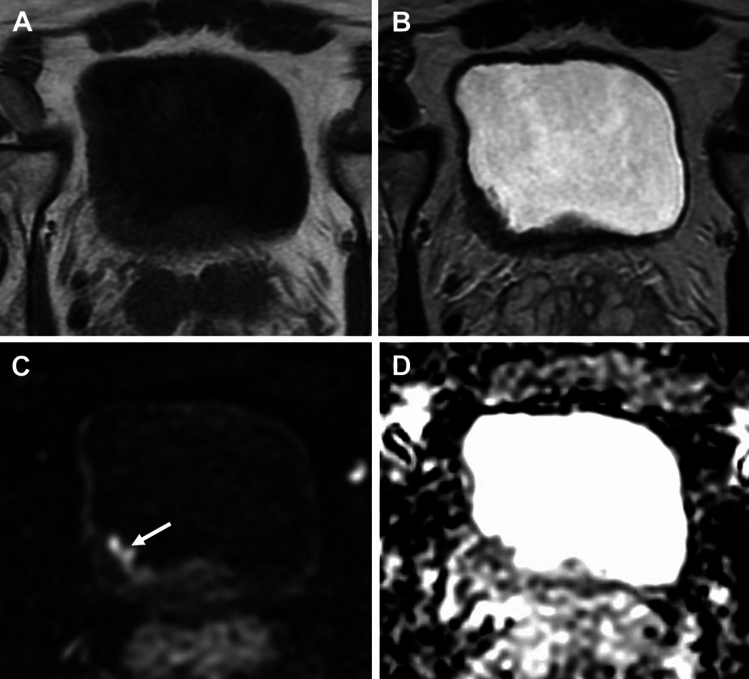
Axial T1WI (A), T2WI (B), DWI (C) and ADC map (D) in a 67-year-old male with urothelial carcinoma who underwent MRI after TURBT shows a 9 mm lesion at the right ureteric orifice post-TURBT (G3pTa). Based on the anatomical sequences this finding could be interpreted as residual disease (A, B). Although this was hyperintense at DWI (arrow) there was no diffusion restriction (C, D) as ADC was not low, and therefore this was interpreted as negative for residual/recurrence disease. Serial cystoscopic biopsies showed no evidence of malignancy
